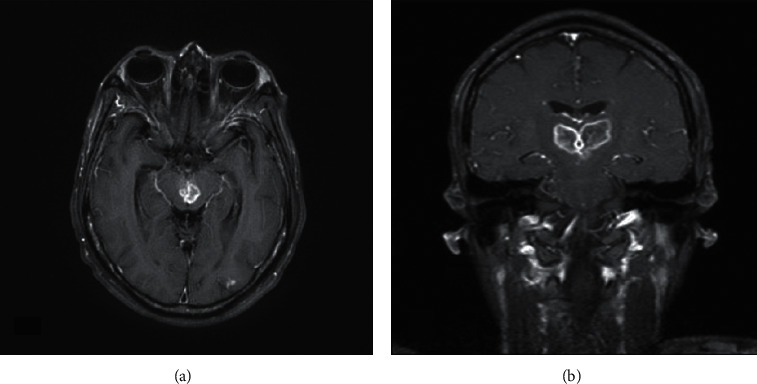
Postcontrast T1 MR images demonstrate vivid contrast enhancement involving the midbrain: (a) axial image and posterior thalami; (b) coronal image.
